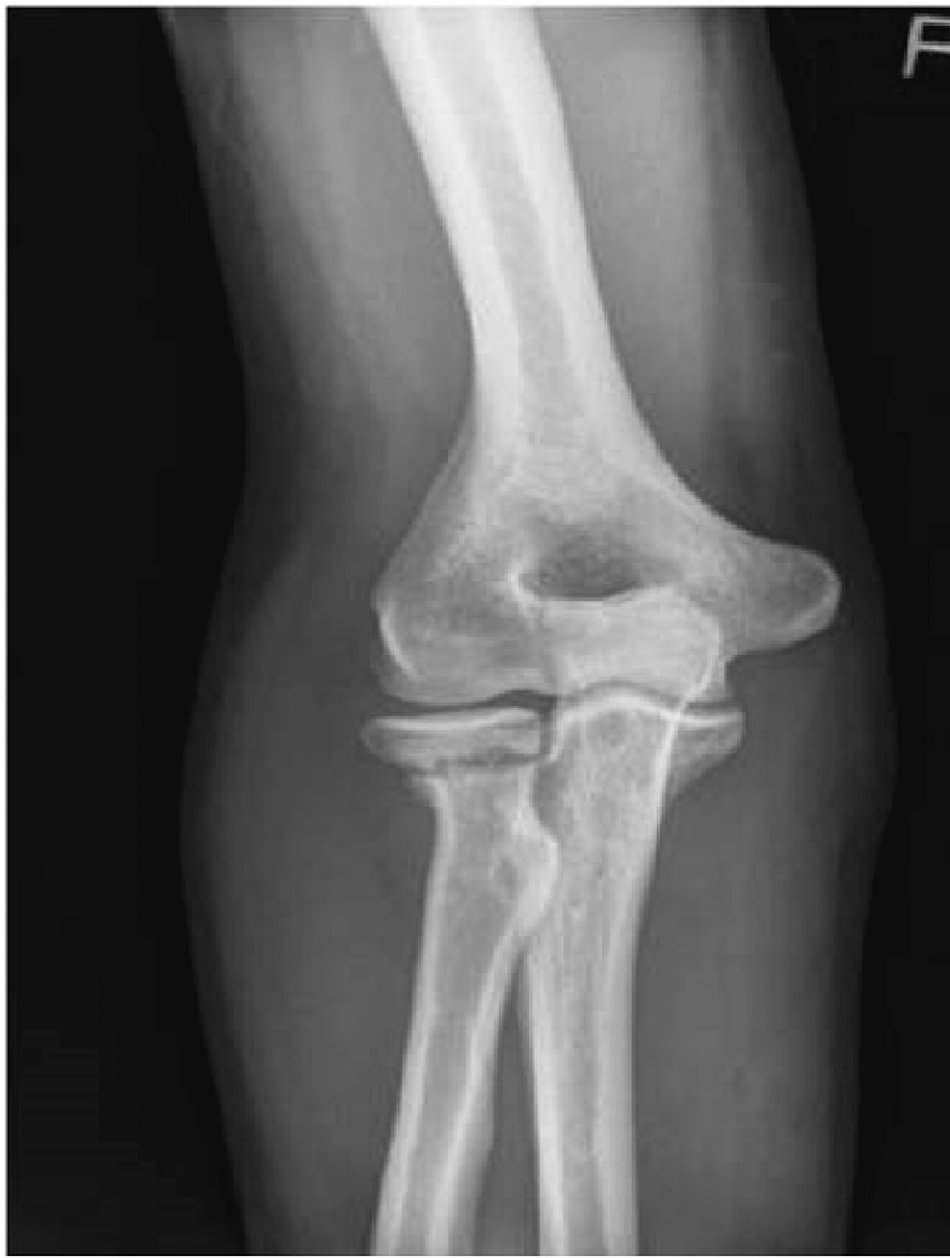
Xray demonstrating an example of radial neck nonunion Reproduced from Pace et al. [23] with permission.
